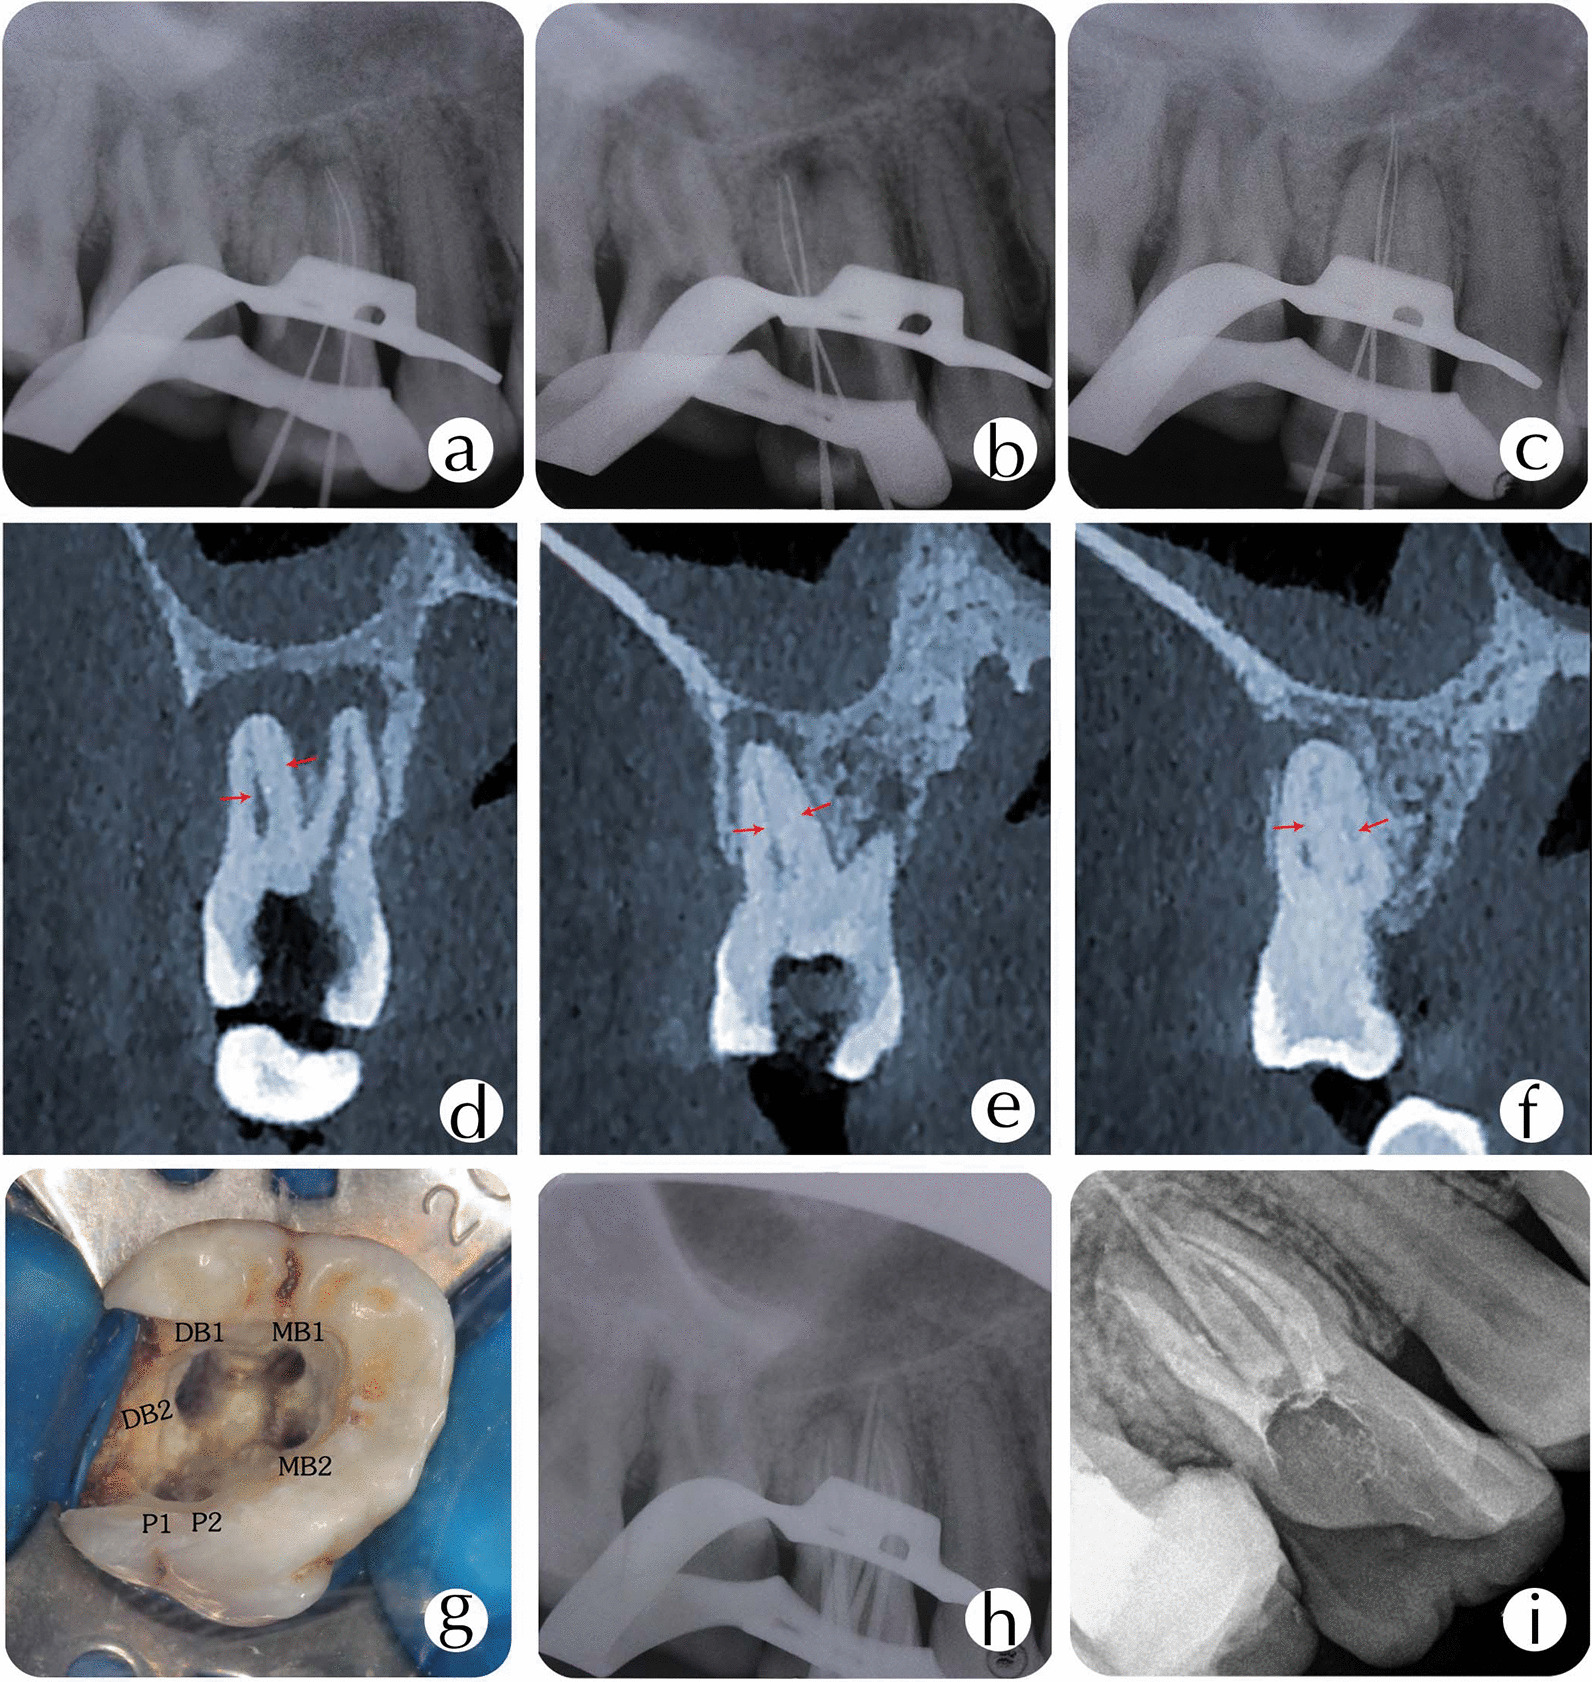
Working length radiograph of tooth #16 in the a mesiobuccal, b distobuccal, and c palatal roots. The MPR views of CBCT showing canal configuration in the d mesiobuccal root canal, e distobuccal root canal, and f palatal root canal. g Access opening showing the six root canal orifices. h Master cone fit. i Post-obturation radiograph
